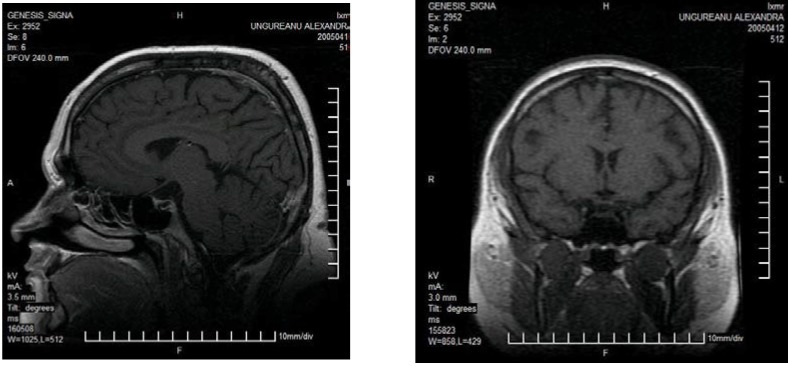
Magnetic Resonance Imaging (MRI) of the Head: normal findings
